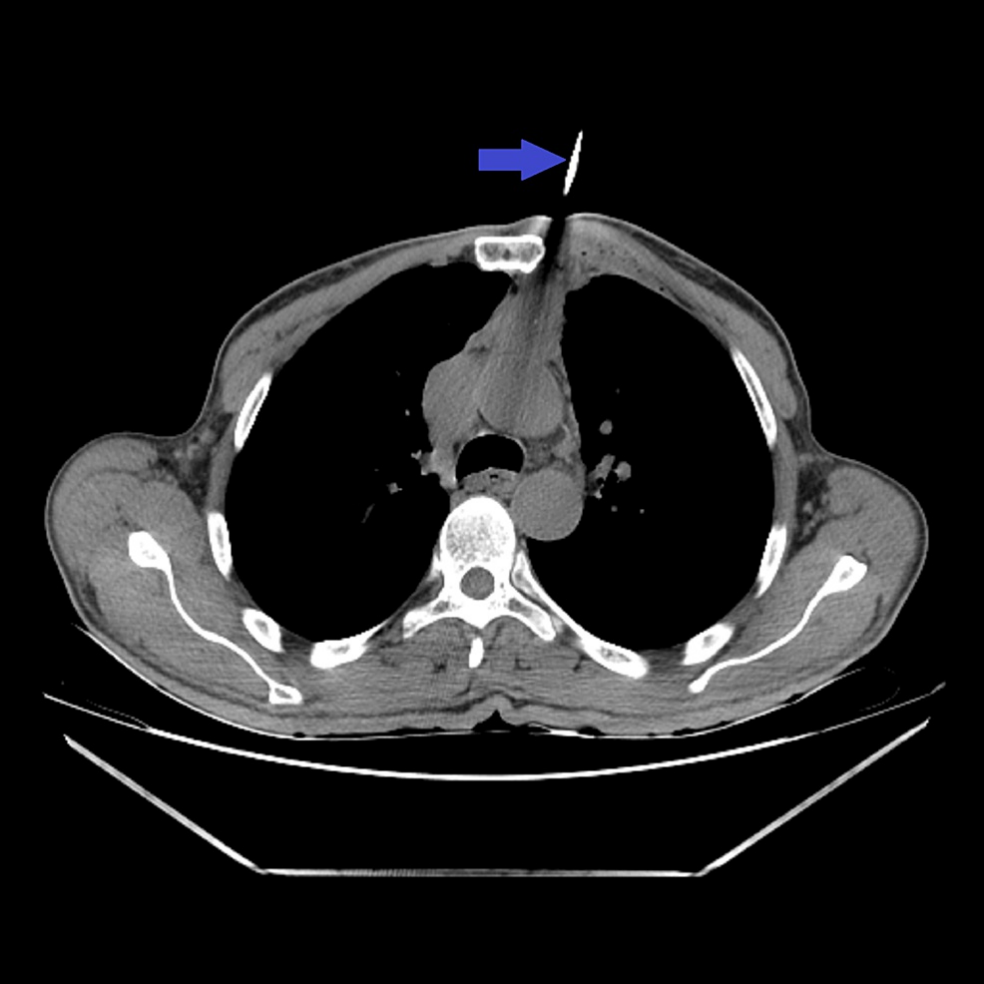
Core needle biopsy being done under CT guidance (blue arrow).
